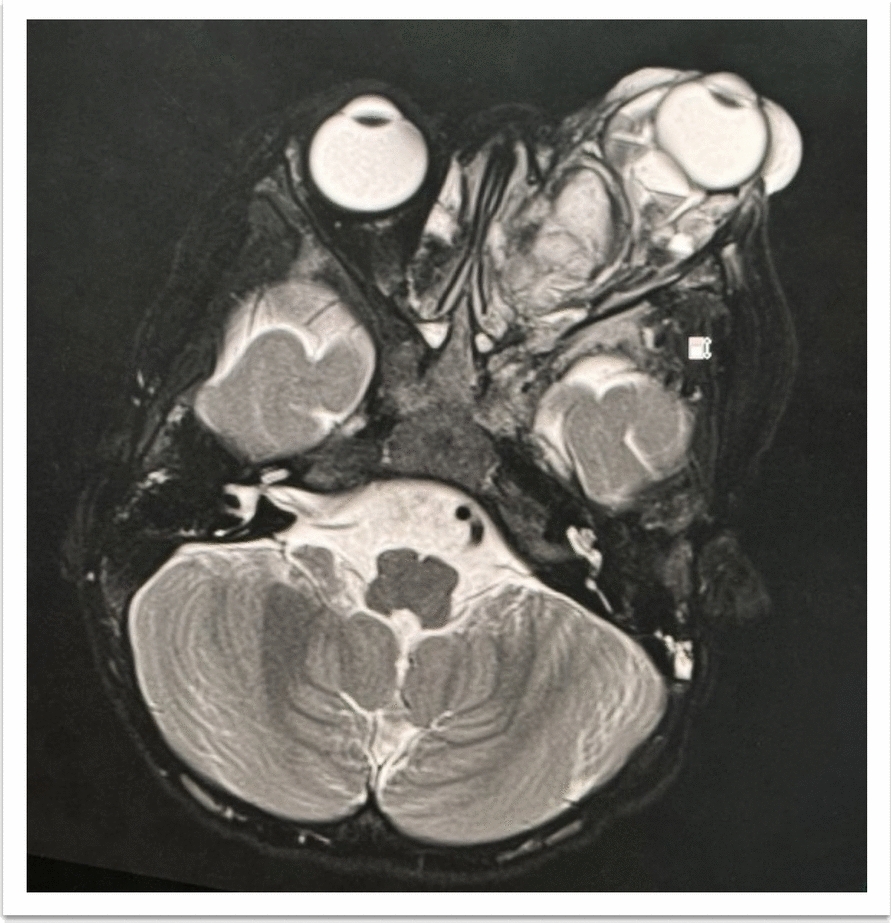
Magnetic resonance imaging showed left extraconal and intraconal orbital masses with fluid levels consisting of blood products with abnormal vessels and dilated superior ophthalmic vein
